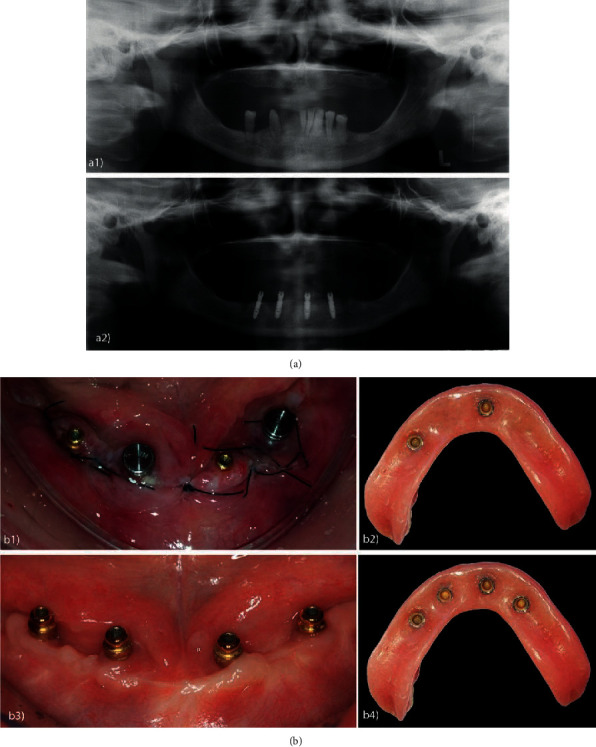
(a) Panoramic X-rays: (a1) preoperative and (a2) immediate postoperative; (b) hybrid occlusal loading: (b1) occlusal view of the O-rings with cylinders in position for capture; (b2) view of the prosthesis base after capturing the O-rings with cylinders of implants 2 and 4; (b3) occlusal view of the attachments prior to capturing implants 1 and 3; (b4) view of the prosthesis base after final capturing.
